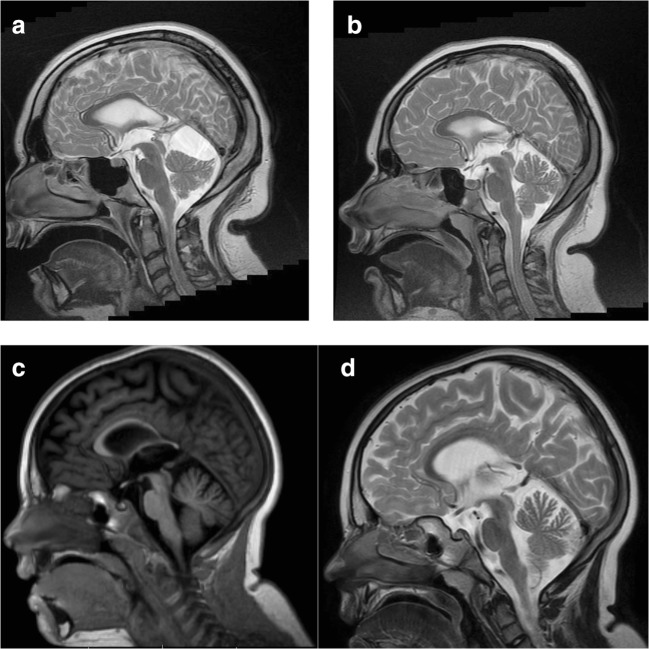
a MRI image of patient 1 (family 1), sagittal section. In T2-weigted image, thinning of the posterior part of corpus callosum is visible as well as an infratentorial cyst modeling the cerebellum. See also brachycephaly due to small brain. b MRI image of patient 2 (family 1), sagittal section. In T2-weighted image, thinning of a posterior part of corpus callosum is visible. See also brachycephaly due to small brain. c, d MRI of patient 3 (family 2), sagittal section. In T1-weigted image, the similar thinning of the posterior part of corpus callosum is visible. In this patient, a relative white matter loss can also be recognized in a T2 sagittal section
